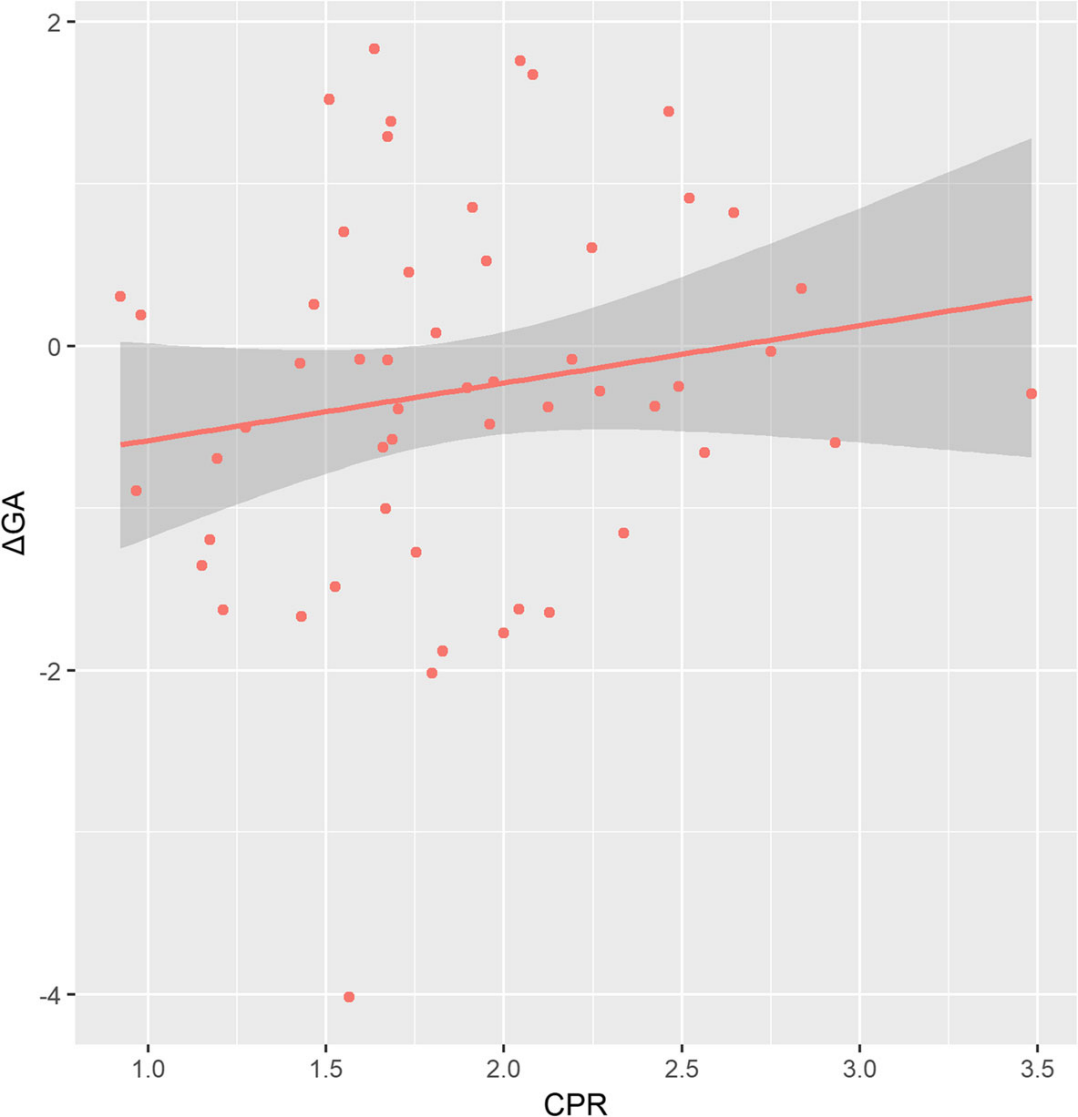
Association between epigenetic age acceleration and cerebroplacental ratio measured during the third trimester. Epigenetic age delta (ΔGA) corresponds to estimated epigenetic age minus chronological age. Thus, ΔGA-positive values reflect epigenetic age acceleration while negative values point out the presence of epigenetic age deceleration. The cerebroplacental ratio (CPR) is calculated as the ratio between the MCAPI and UAPI. Both variables were significantly correlated when adjusting for sex, chronological gestational age, birth weight, and gestational age at ultrasound
